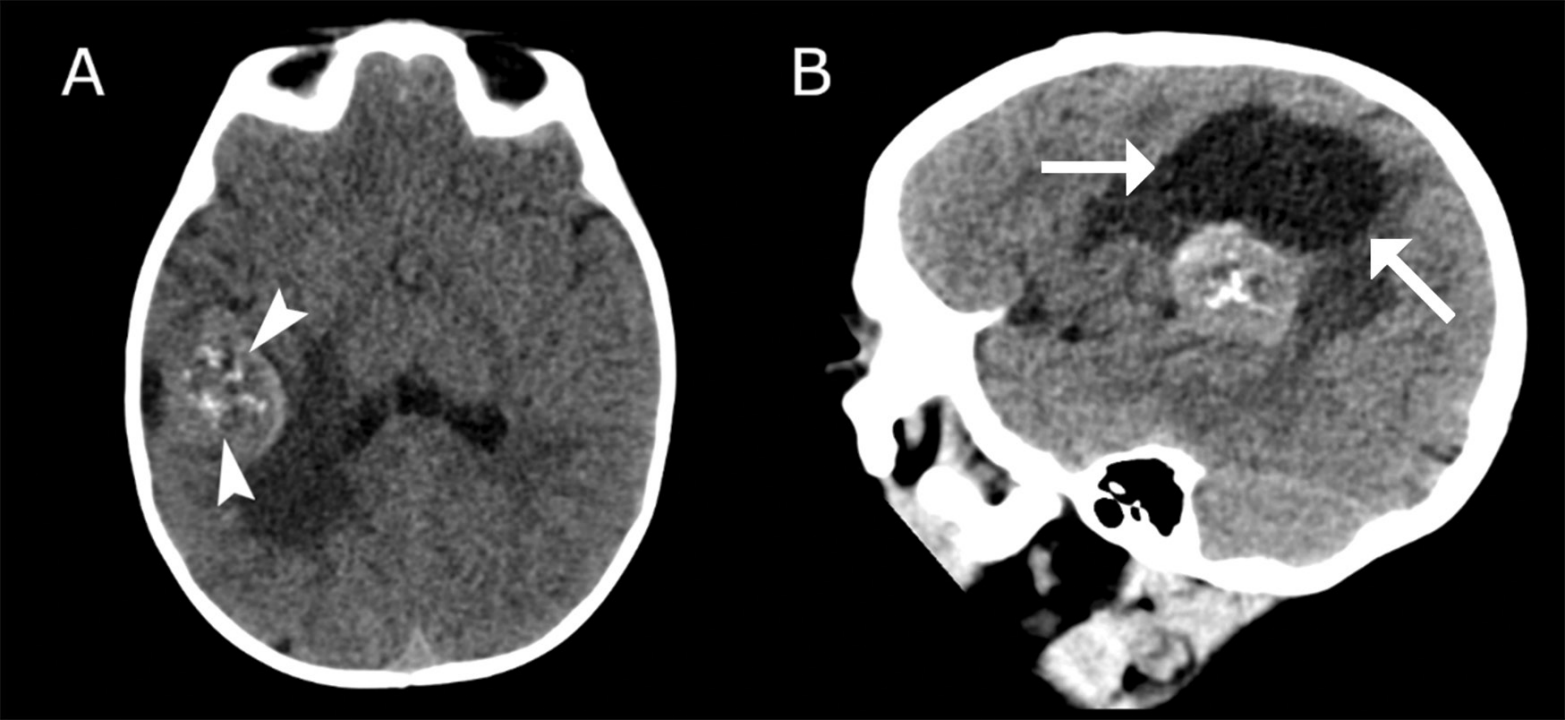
Imaging findings on non‑contrast CT brain. (A) Axial slices show a lesion in the right cerebral hemisphere with perilesional edema causing mass effect on the right lateral ventricle. Coarse calcifications are seen centrally within the lesion (arrowheads). (B) Sagittal slices show a large cystic component superiorly to and in close relationship with the lesion (arrows).
